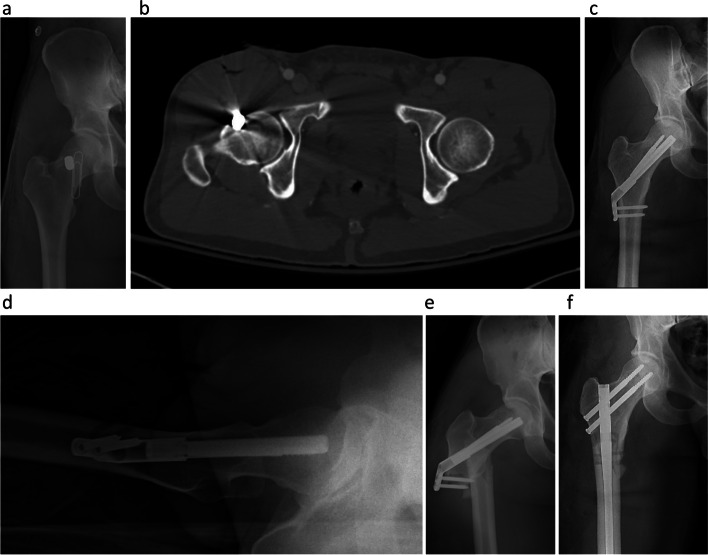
a 41-year-old male presented to our institution after a gunshot wound to his right groin. Panel a is an AP radiograph of the right hip showing a foreign body overlying the femoral neck. Paper clip denotes the entry wound of the missile. Panel b is an axial cut from computed tomography of the right hip showing an intracapsular missile with disruption of the anterior cortex of the femoral neck. Panel c is a postoperative anteroposterior and d lateral radiograph of the right hip after implantation of a femoral neck system showing unfilled screw holes in the lateral cortex. Panel e is an AP radiograph of the right hip 17 days post-operatively revealing a peri-implant subtrochanteric fracture. He was treated successfully with a piriformis entry recon nail shown in panel f
